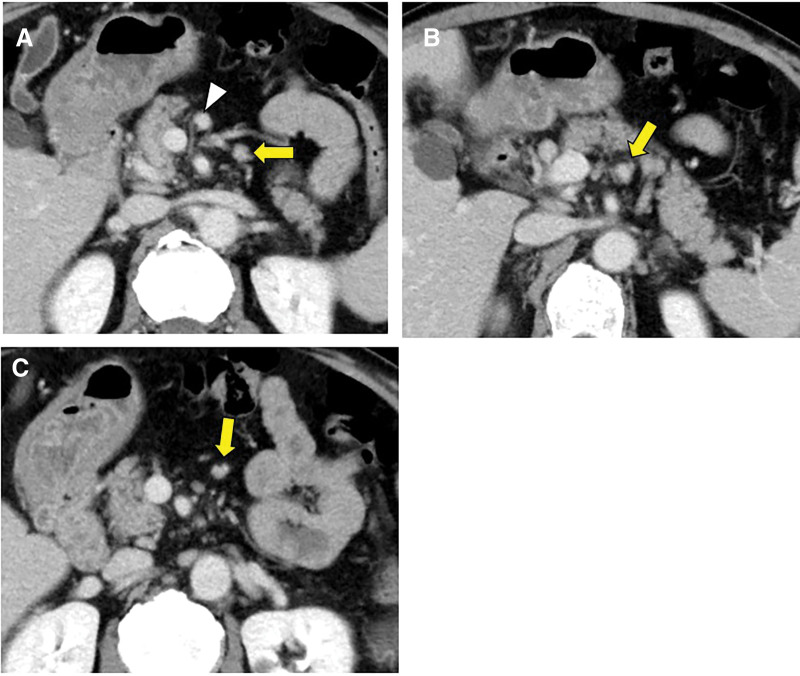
Contrast-enhanced CT obtained 3 years prior to diagnosis for evaluation of chronic cough revealed a 10-mm enhancing lesion in the pancreatic body (white arrowhead) (A) and 3 nodules in the transverse mesocolon (yellow arrows) (B and C).
